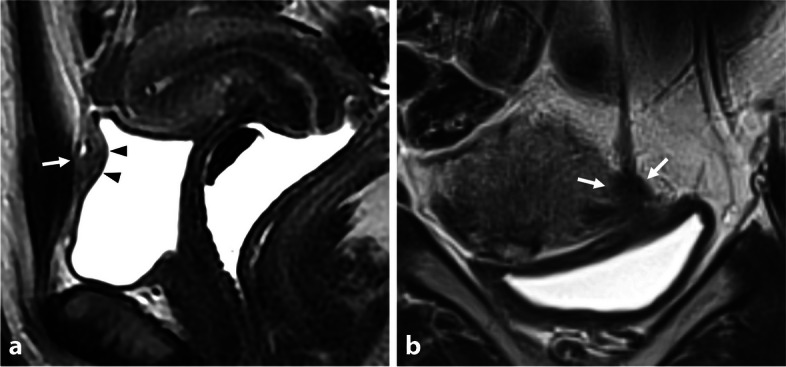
Fibrous remnant of the urachus insertion in two distinct women of reproductive age. a Sagittal and (b) coronal T2-W MR images show a pseudonodular or triangular T2-hypointense medial structure of the urinary bladder apex at the urachus insertion (arrows). Note the absence of abnormality of the urinary bladder muscular layer (arrowheads), or hemorrhagic foci on T1-WI (no shown)
